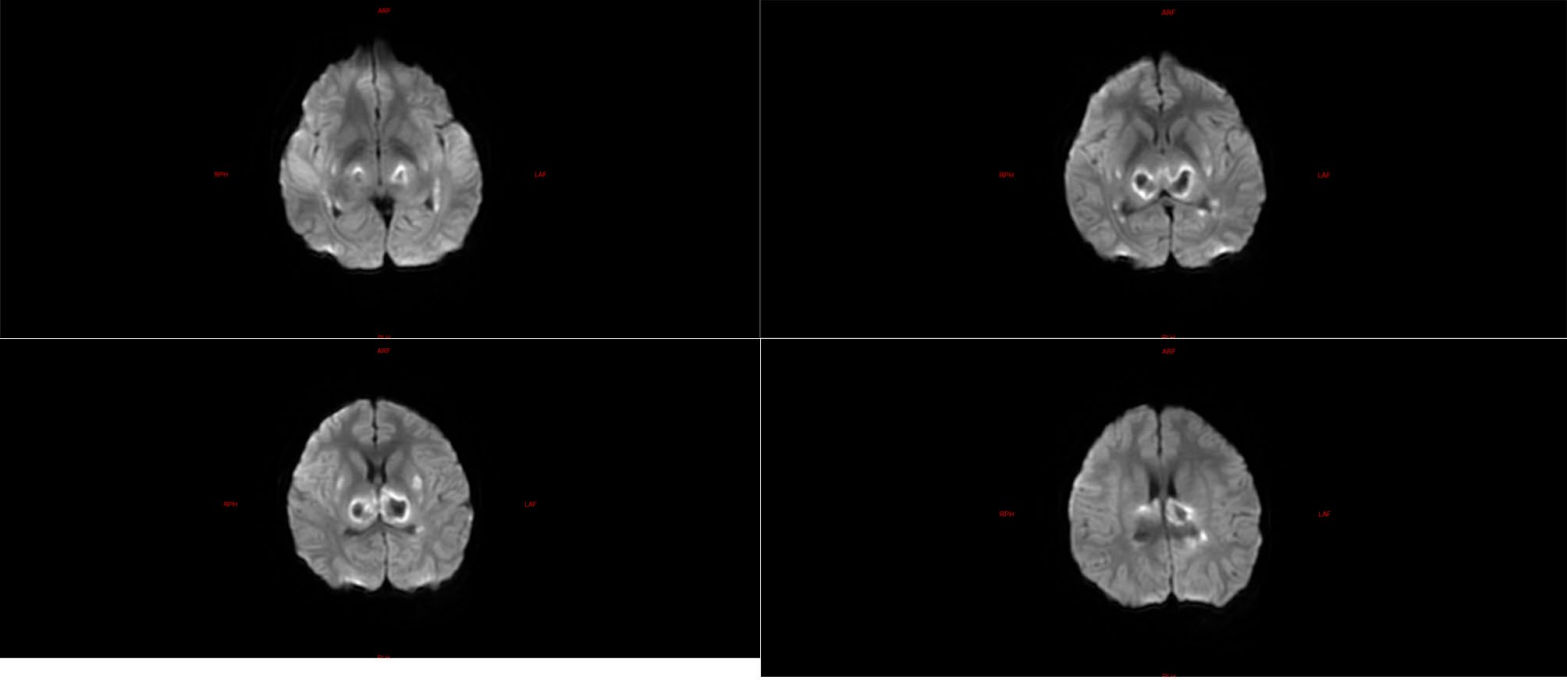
Brain MR DWI sequence of case 4, showed bilateral basal ganglia, thalamus, and white matter adjacent to the posterior horn of the lateral ventricle with symmetrical diffuse high signal, and a low signal was seen in the bilateral thalamic lesions, and hemorrhage was considered. The patient presented with typical features of necrotizing encephalopathy, exhibiting significant consciousness impairment and irregular respiratory rhythm upon admission. Following treatment, the patient was transferred from the ICU, but later experienced cognitive regression and impairment in motor function
